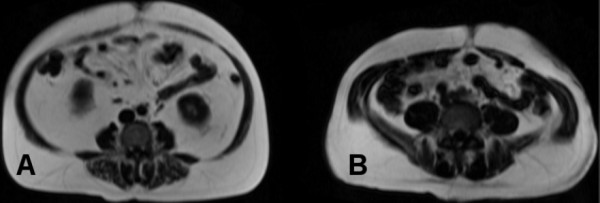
Axial MR images from two volunteers. One volunteer had an exceptionally large amount of VF (A) but smaller amount of SF than a volunteer with a reasonable amount of VF (B). The relative amount of conductive tissue (muscle) compared with poorly conductive fatty tissue is so small in (A) that the BELA could not differentiate VF from VF+SF.
